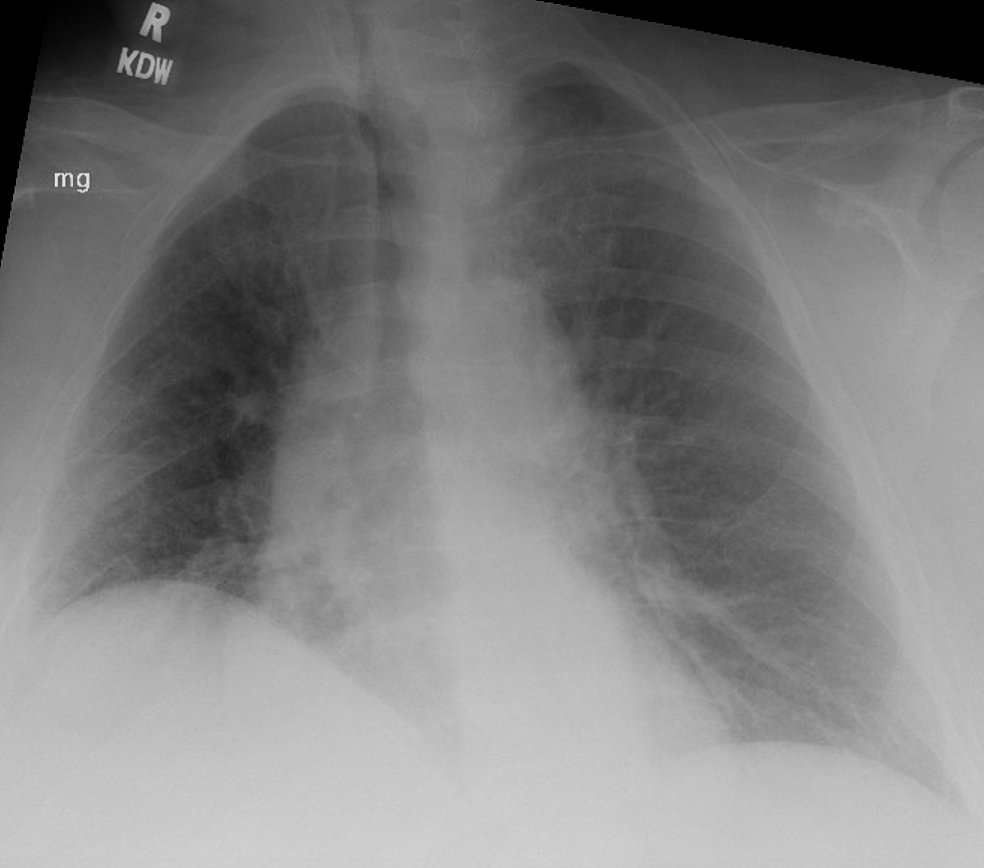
Initial chest X-ray The chest X-ray reveals interstitial opacities predominantly of the lower lung, suggestive of pulmonary edema. No obvious pulmonary masses are seen.
